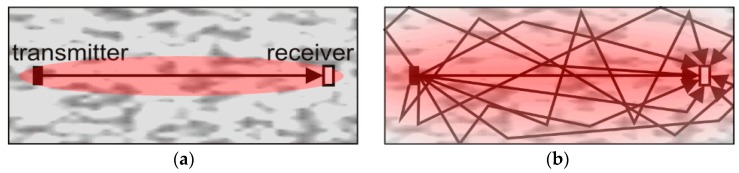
Principle of ultrasonic transmission measurements, propagation paths, and areas of influence (red). (a) Direct wave (time of flight); (b) multiple scattering (coda).
